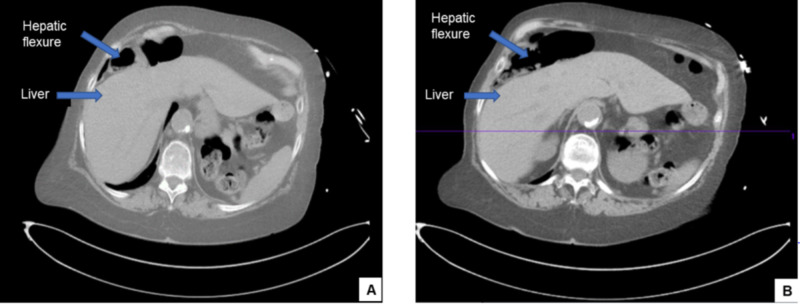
(A, B) CT scans of the abdomen reveals the interposition of hepatic flexure anteriorly in front of the liver up the dome of the diaphragm (Chilaiditi sign).
